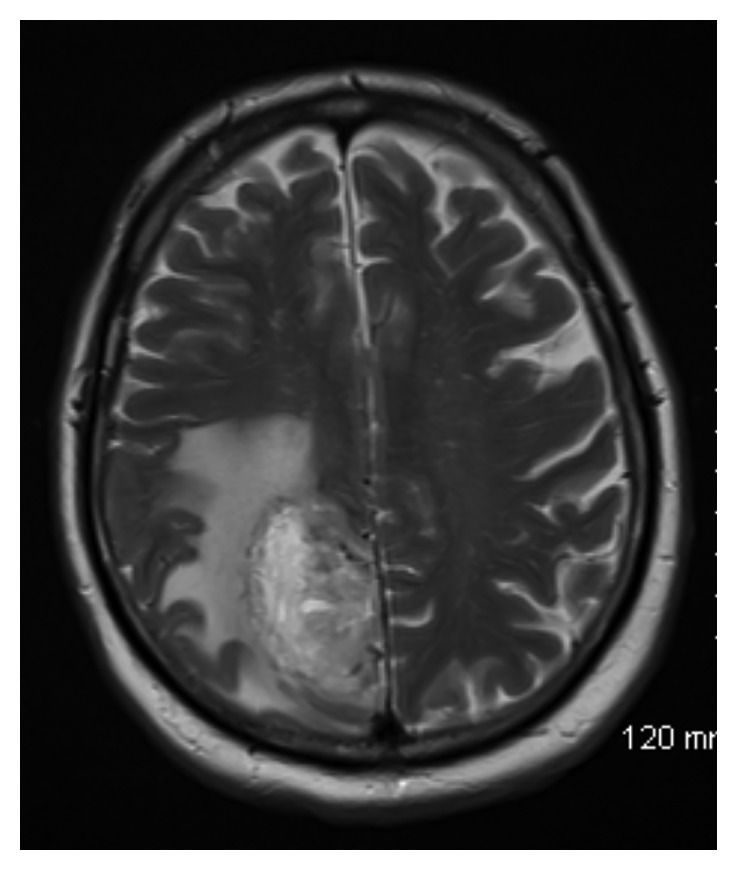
Axial T2-weighted image demonstrating heterogeneous mass in the right parasagittal parietal lobe with extensive surrounding vasogenic edema.
